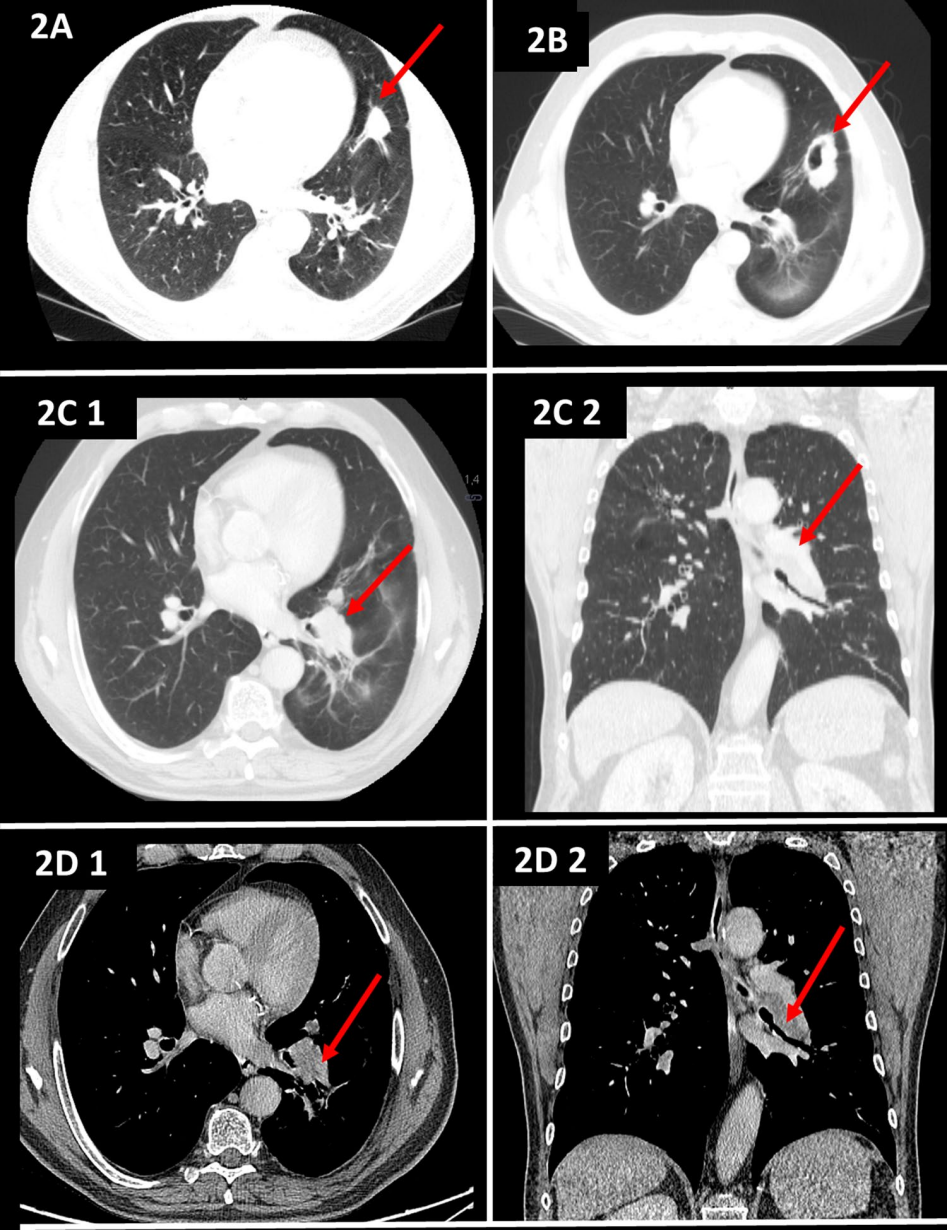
a High-resolution CT image revealing a large nodule in the lower lobes. b High-resolution CT image revealing a cavitated nodule. c CT pulmonary window revealing enlarged lymph nodes in the hilus of the left lung that impress the bronchi to the lower lobe, which, combined with the presence of subpleural nodules, aroused suspicion of a proliferative process (2C1 axial scan, 2C2 coronal scan). d CT soft tissue window revealing a pathological mass in the hilus of the left lung (2D1 axial scan, 2D2 coronal scan)
